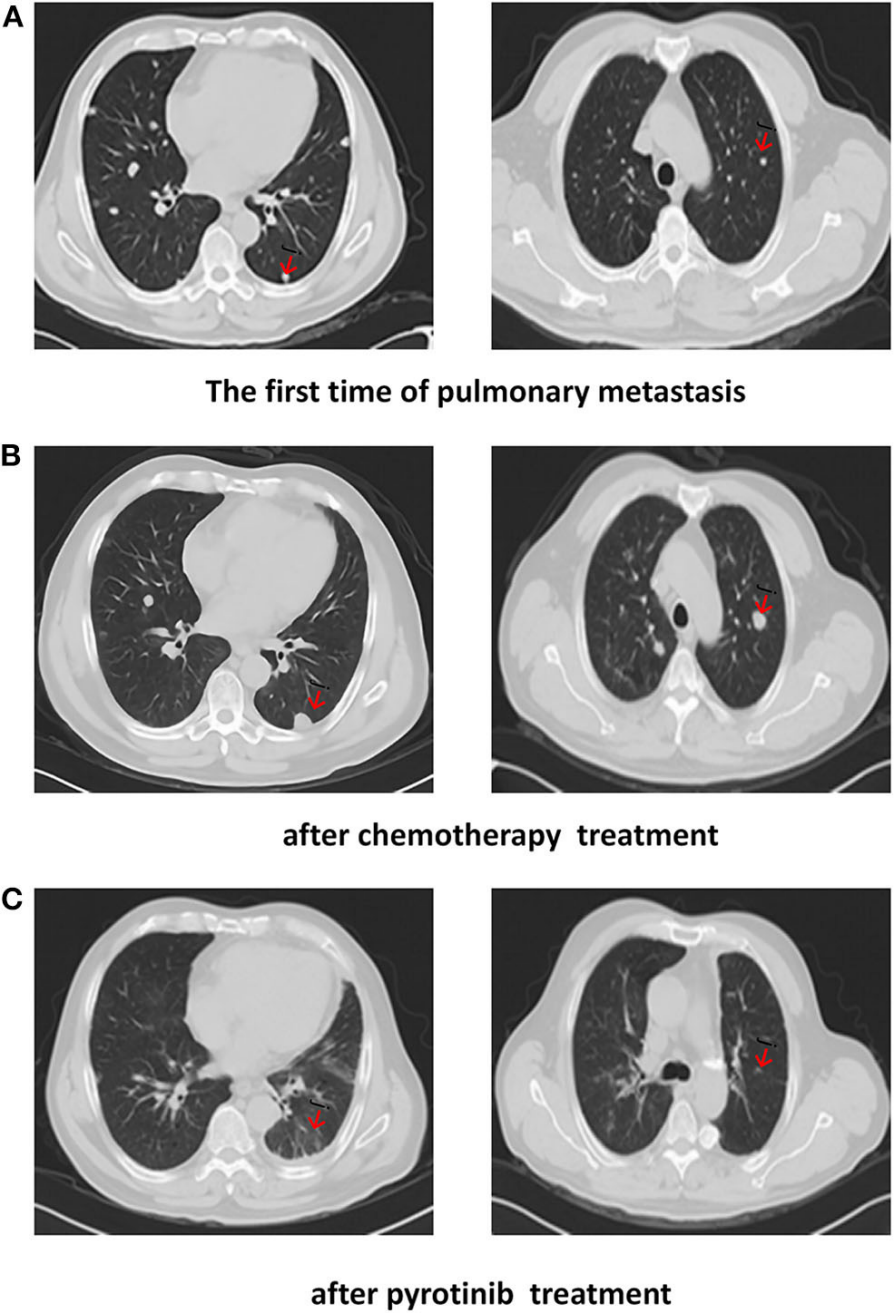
CT scans show: (A) The first time of pulmonary metastasis; (B) after chemotherapy treatment; (C) CT of the chest showed a complete response after months of pyrotinib treatment.
